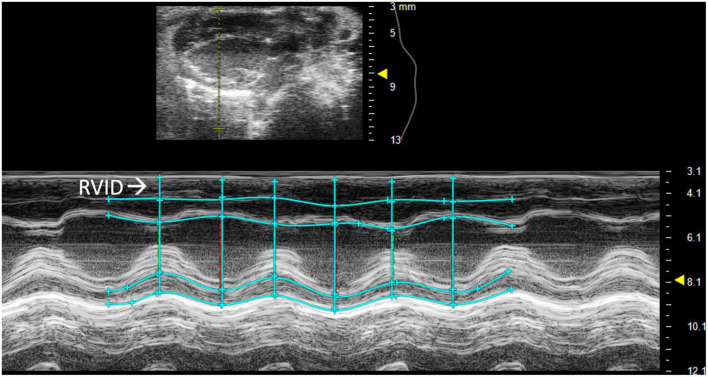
Right ventricular internal diameter (RVID), a measurement of the right ventricle during systole and diastole, is obtained by analyzing parasternal long axis (PLAX) view images in M-mode.
